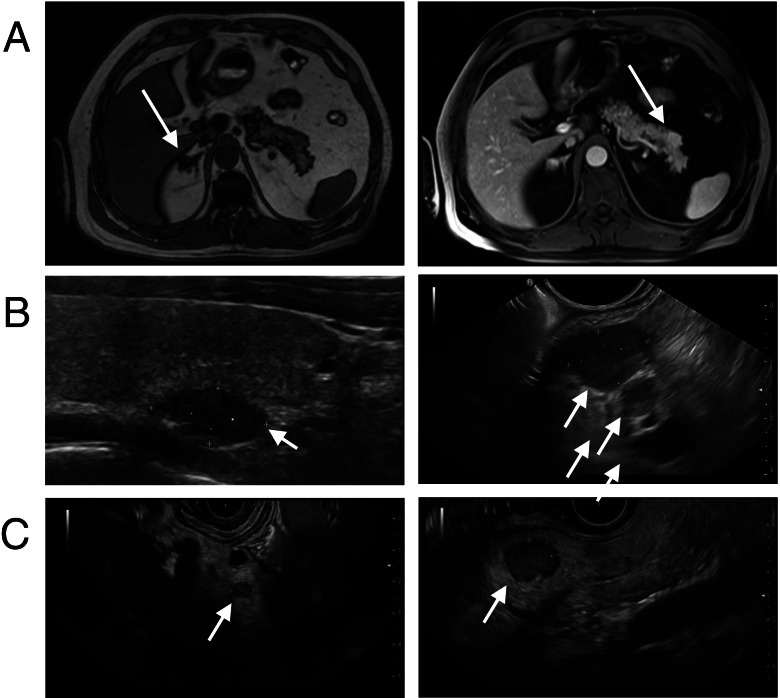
Imaging in the family members of the index patient. Imaging is showing adrenal and parathyroid adenomas and hormone-inactive NET of the pancreas. (A) 58-year-old father. MRI abdomen in-phase (IP) showing an adenoma of the right adrenal gland (left) and out-of-phase (OOP) MRI showing one lesion in the pancreatic tail (right). (B) 19-year-old brother. Ultrasound of the neck showing a right caudal parathyroid adenoma (left) and EUS with two neighboring lesions in the pancreatic tail (right). (C) 26-year-old sister. EUS showing multiple masses of the pancreas
